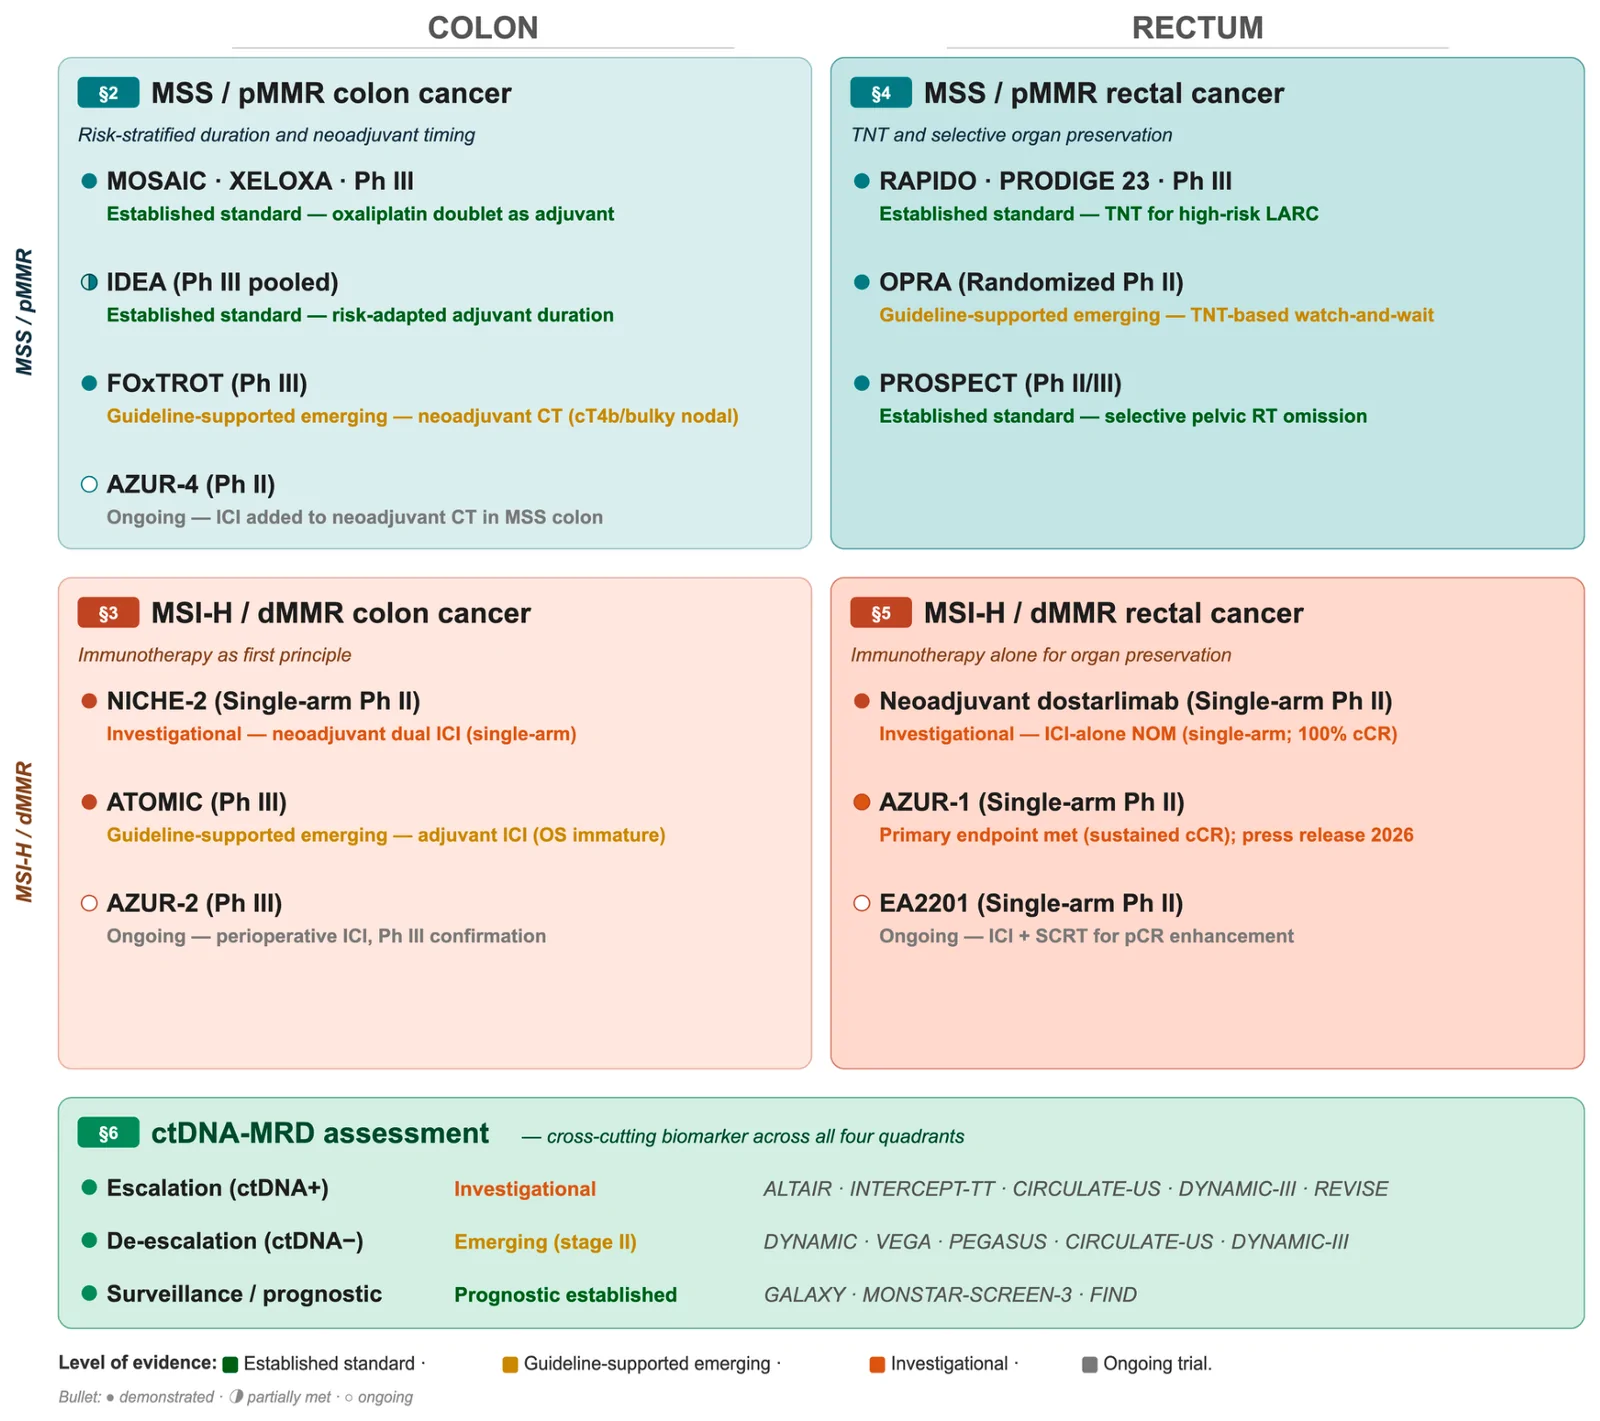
Four-quadrant framework for perioperative management of colorectal cancer, stratified by mismatch repair status and primary site. Trials shown: MOSAIC [2,12]; NO16968/XELOXA [3]; IDEA [4]; FOxTROT [13,14]; NICHE-1 [9]; NICHE-2 [10,15]; NICHE-3 [16]; ATOMIC [17]; RESET-C [18]; AZUR-4 [19]; PRODIGE 23 [6,20]; RAPIDO [5,21]; OPRA [7]; PROSPECT [8]; cohort data for MSI-H/dMMR rectal cancer [11,22]; AZUR-2 [23].
